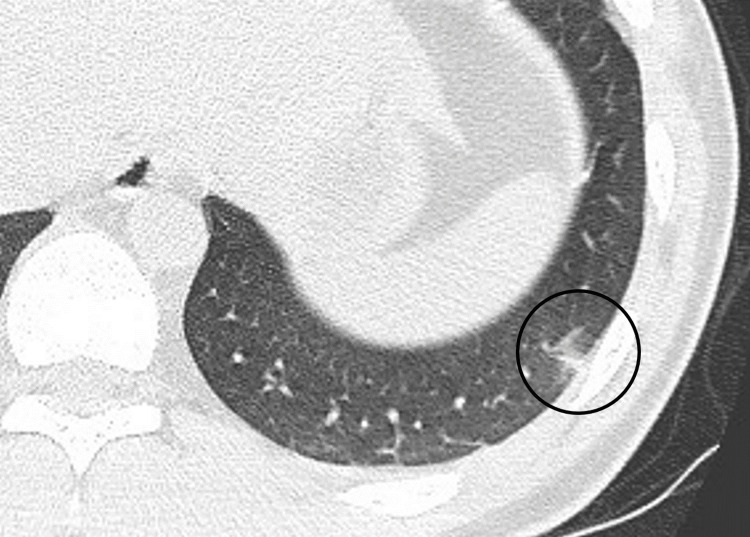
CT revealed a frosted shadow in the lower lobe of the left lung. CT: computed tomography
